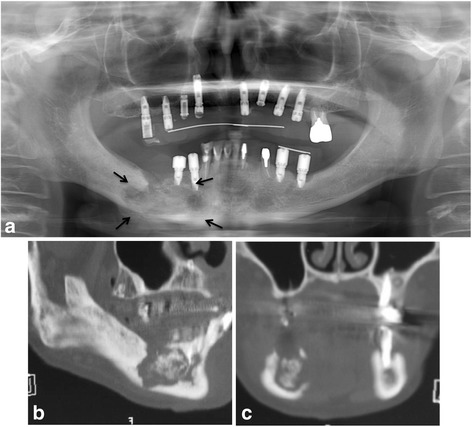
a Panoramic radiograph showing the sequestrum separation after 5 months of teriparatide therapy (*arrows*). b Sagittal CT view. c Coronal CT view
